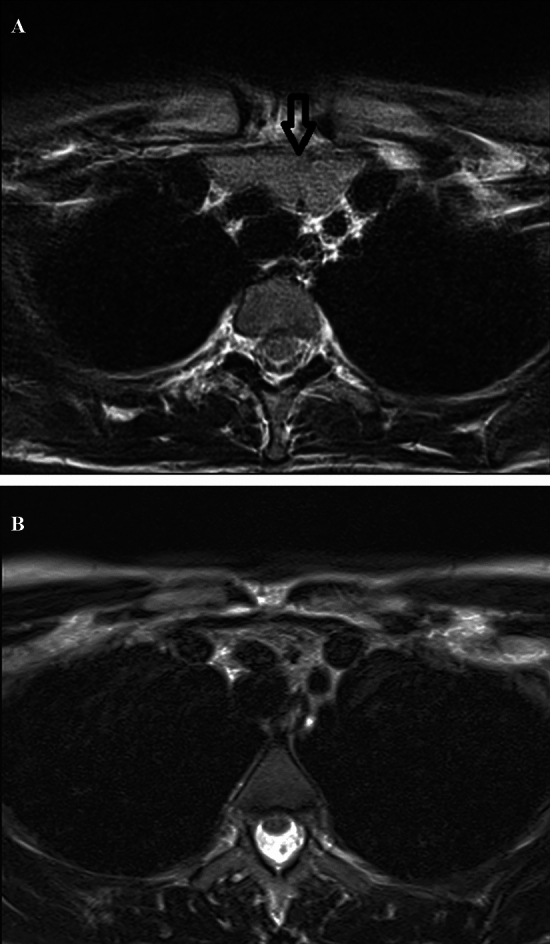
Representative case of GD-associated TH (the same patient of Fig. 2) evaluated by thoracic MRI, at baseline (a) and after achieving stable euthyroidism (b).a 29-year-old woman with GD-associated TH (the same patient of Fig. 2): at diagnosis, axial high-resolution T2 image demonstrated a well lobulated 50 mm (9.8 mL) soft tissue mass with homogeneous signal intensity in the mediastinal prevascular space extending superiorly to the level of the left brachiocephalic trunk (arrow), b that disappeared after 6 months of methimazole-based therapy under stable euthyroidism. TH, thymic hyperplasia; GD, Graves’ disease, MRI, magnetic resonance imaging
